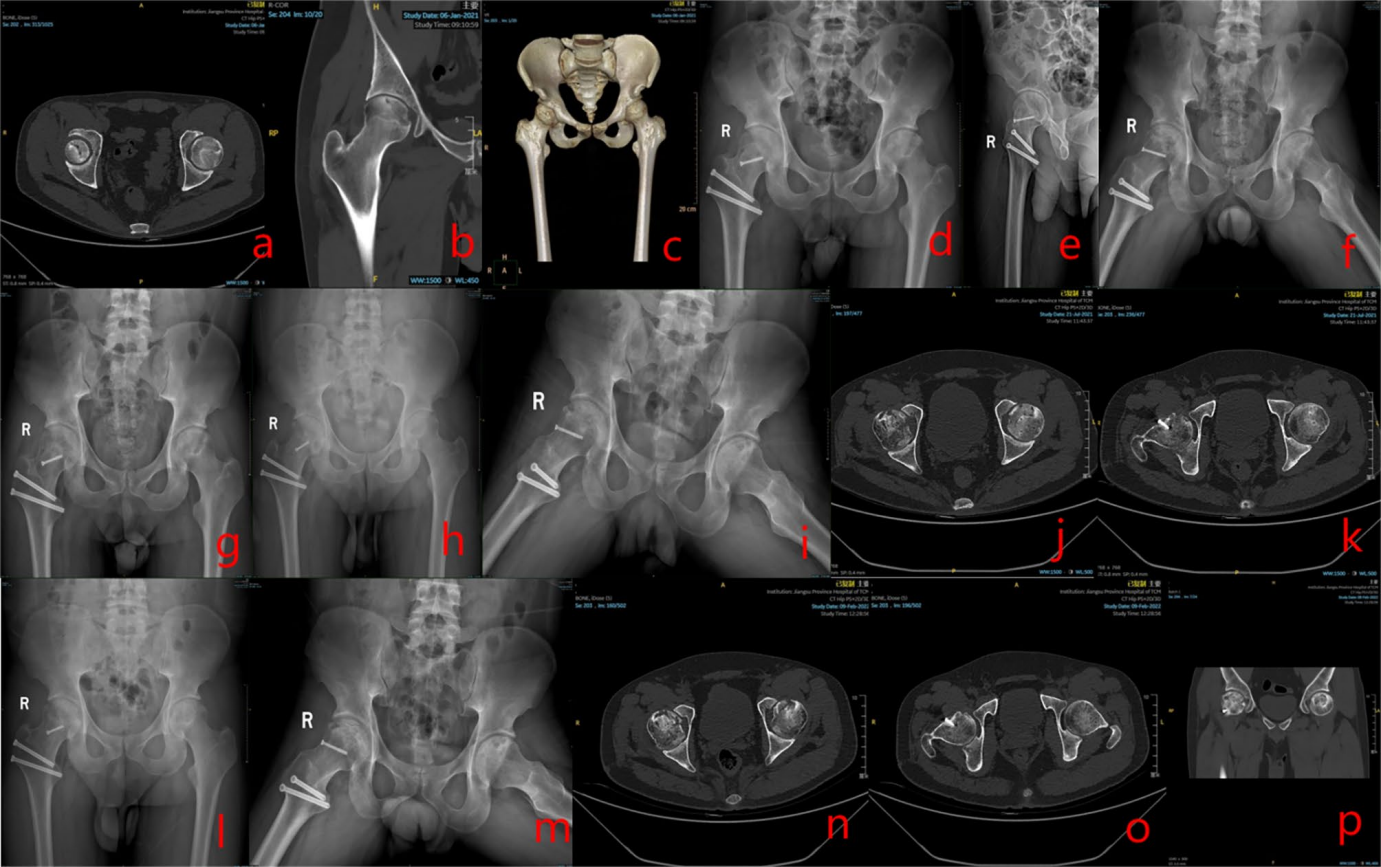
Preoperative (a–c), postoperative (d–e), 3 months postoperative (f–g), 6 months postoperative (h–k), and 12 months postoperative (l–p) X-rays, CT and 3D reconstruction.
